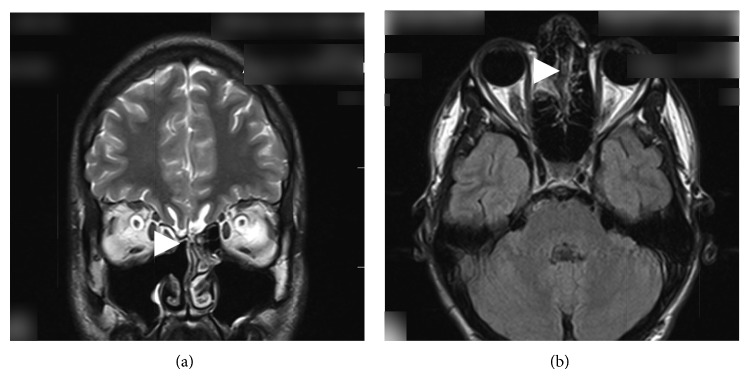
((a)-(b)) Control MRI after 8 months. The defect in the skull base is sealed; there is no more CSF leakage and no brain protrusion.
